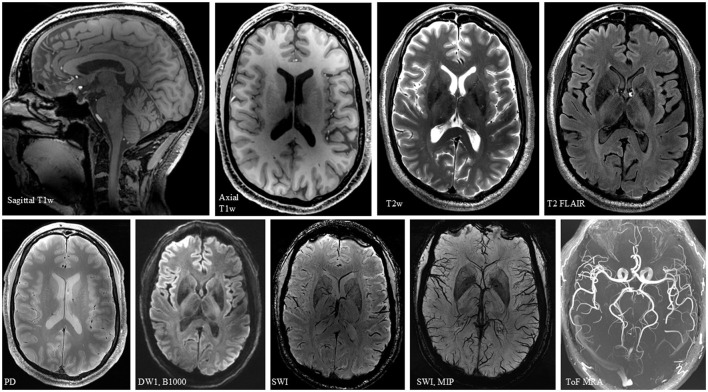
Images of the brain obtained from a subject scanned with the 8Tx64Rx (CP). These include a sagittal T1-weighted acquisition through the midline structures; 3D T_1_-weighted, T_2_ TSE, T_2_ FLAIR, PD, and DWI (*b* = 1,000 s/mm^2^), SWI and SWI Minimum Intensity Projection (MIP) axial acquisitions acquired at a plane through the level of basal ganglia, insula and corona radiata; and TOF MRA (MIP) through the Circle of Willis.
